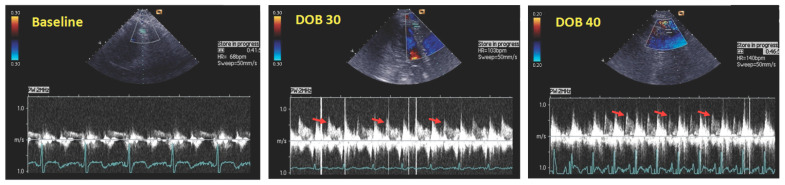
Characteristic diastolic coronary flow velocity profile (“finger-tip” phenomenon: red arrows) in the distal segment of left anterior descending artery, below the myocardial bridging, obtained by transthoracic Doppler echocardiography during high-dose dobutamine infusion (DOB: 30–40 µg/kg/min). This phenomenon is characterized by an abrupt coronary flow acceleration followed by rapid deceleration during early diastole and mid-to-late diastolic flow plateau. Adapted with permission from Aleksandric et al. [54].
